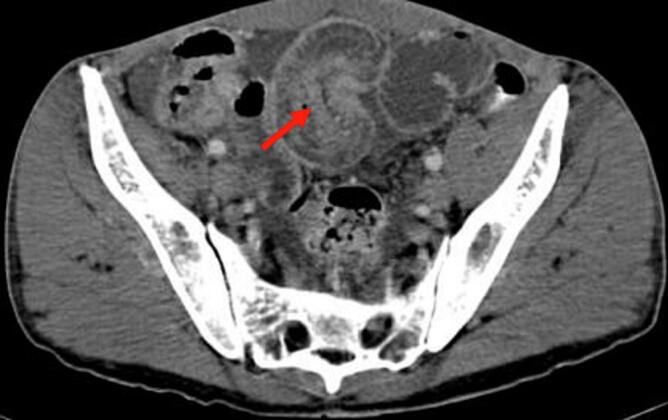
An abdominal CT scan showing the intestinal telescoping image with distention of the downstream segment (red arrow). (For interpretation of the references to colour in this figure legend, the reader is referred to the web version of this article.)
